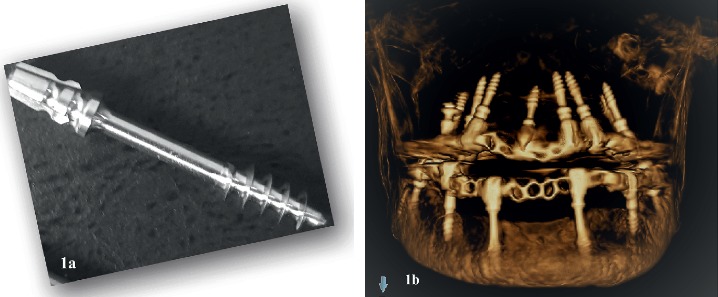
(a) BCS® basal implant design. (b) A three-dimensional cone-beam computed tomography image shows the anchorage of the BCS® implants within the basal bone in patients presented with a severely resorbed alveolar ridge.
